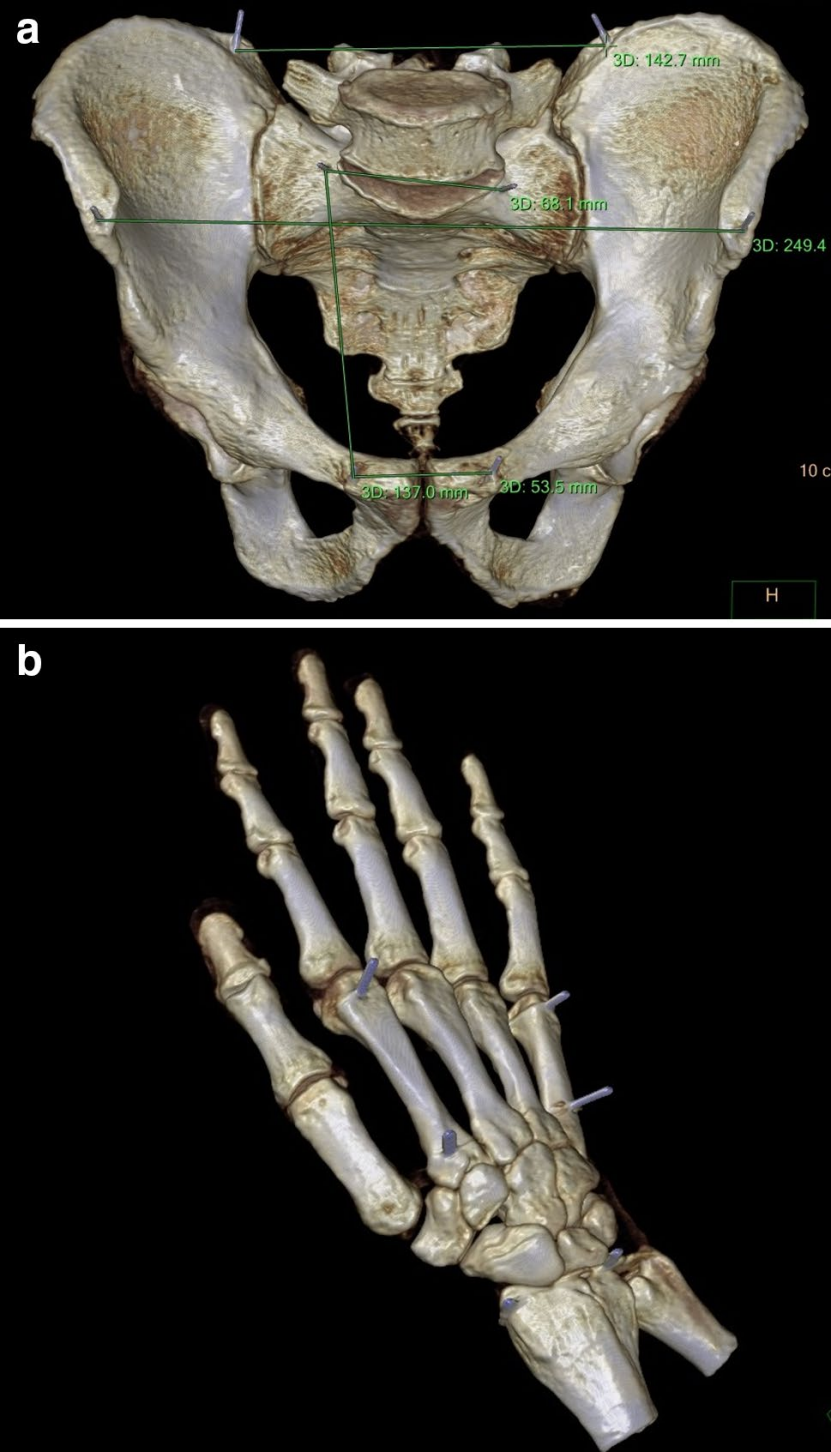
In this figure a 3D model of a pelvis after CT-scanning is seen with all measurements between the five marker points performed on the Philips Intellispace Portal
